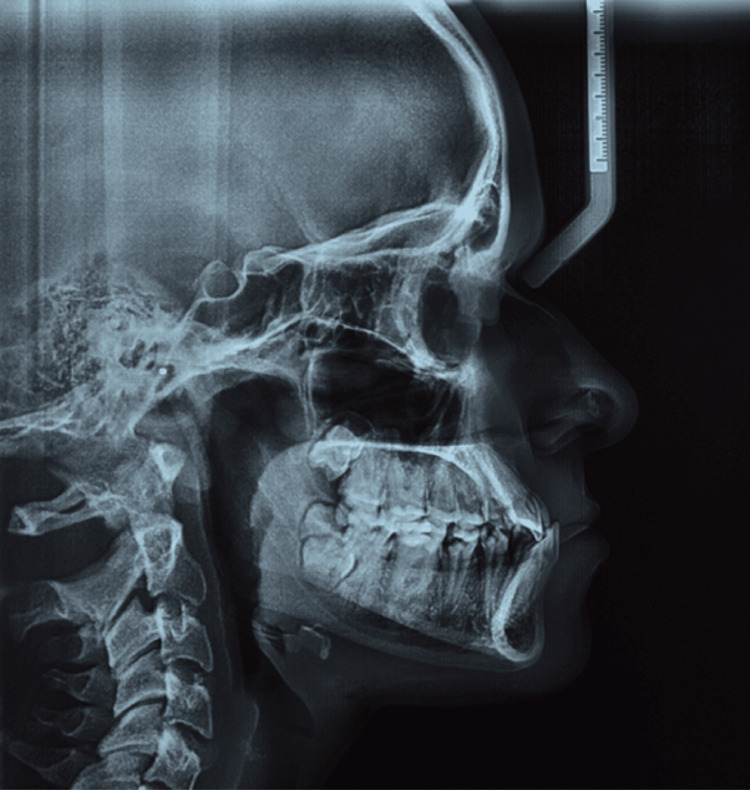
Pre-treatment lateral cephalometric analysis Lateral cephalometric radiograph depicting the initial skeletal pattern and dentoalveolar relationships.
